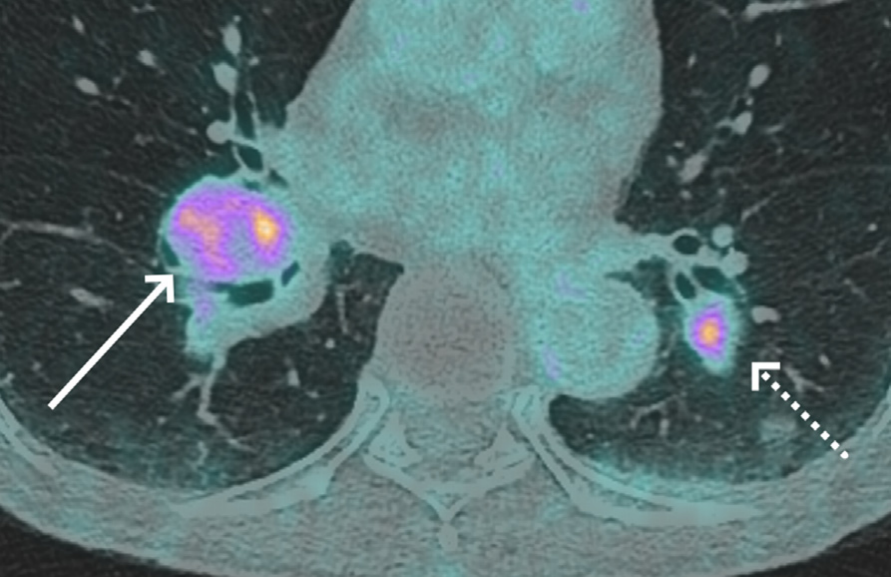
PET-CT showing hyperactivity in the hilar lymph nodes (arrow) and within the segmental embolism (dotted arrow), proving its tumoral nature.
